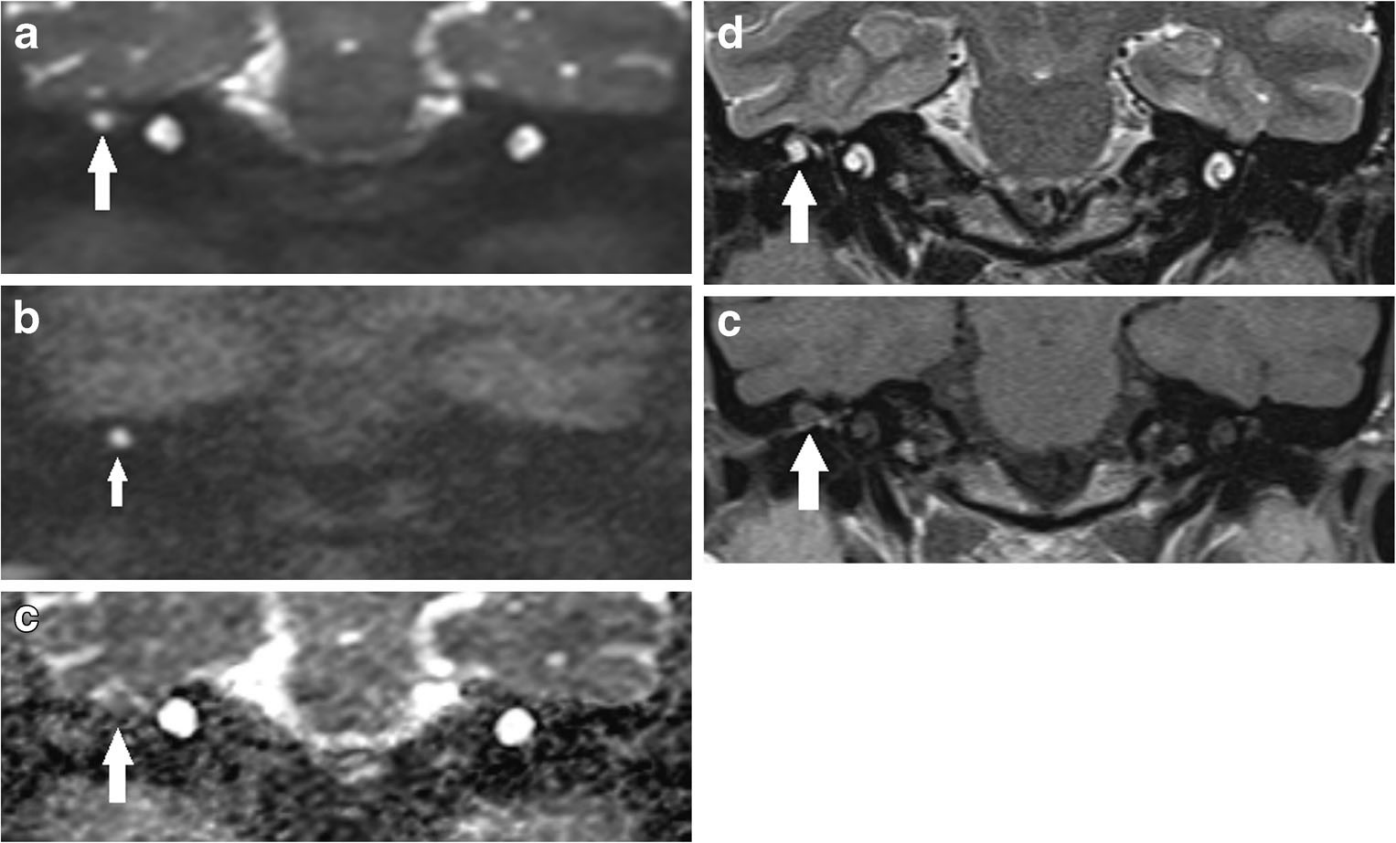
DWI appearance of a small residual cholesteatoma confirmed on second-look surgery. The a b0 and b b1000 image shows a small 5 mm high signal cholesteatoma (*white arrow*) at the right epitympanum. It returns low signal on c the corresponding ADC map, high signal on d the T2 weighted image and low signal on e the non-contrast enhanced T1 weighted image
